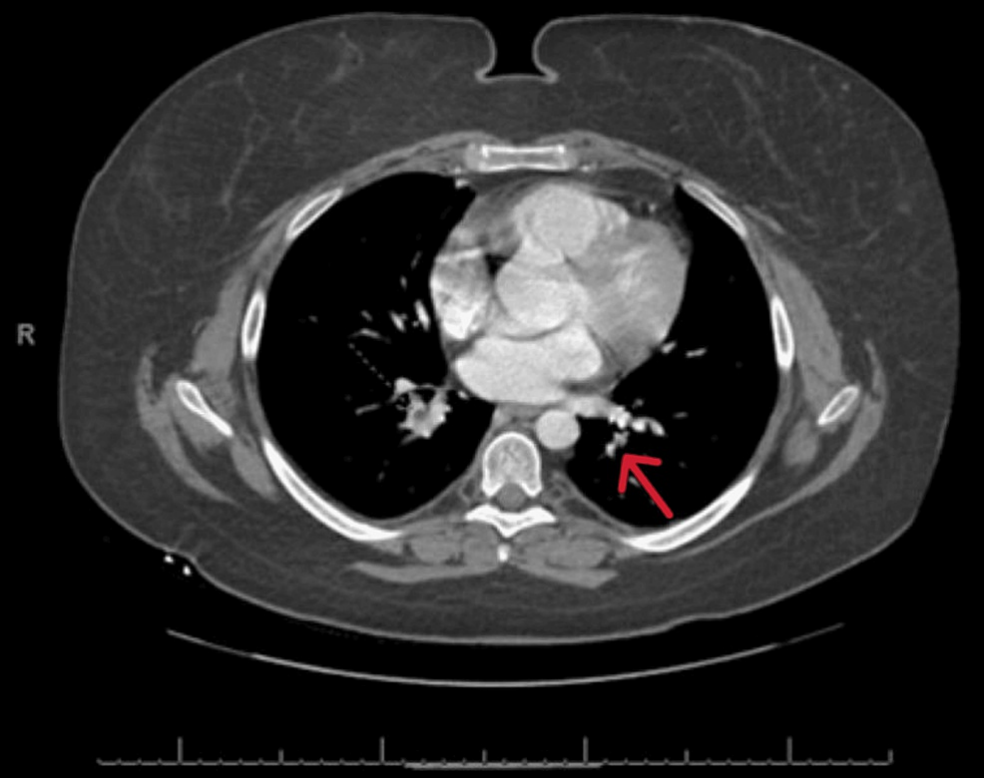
CT scan of the chest (axial view) with contrast showing the left pulmonary embolism (red arrow).
